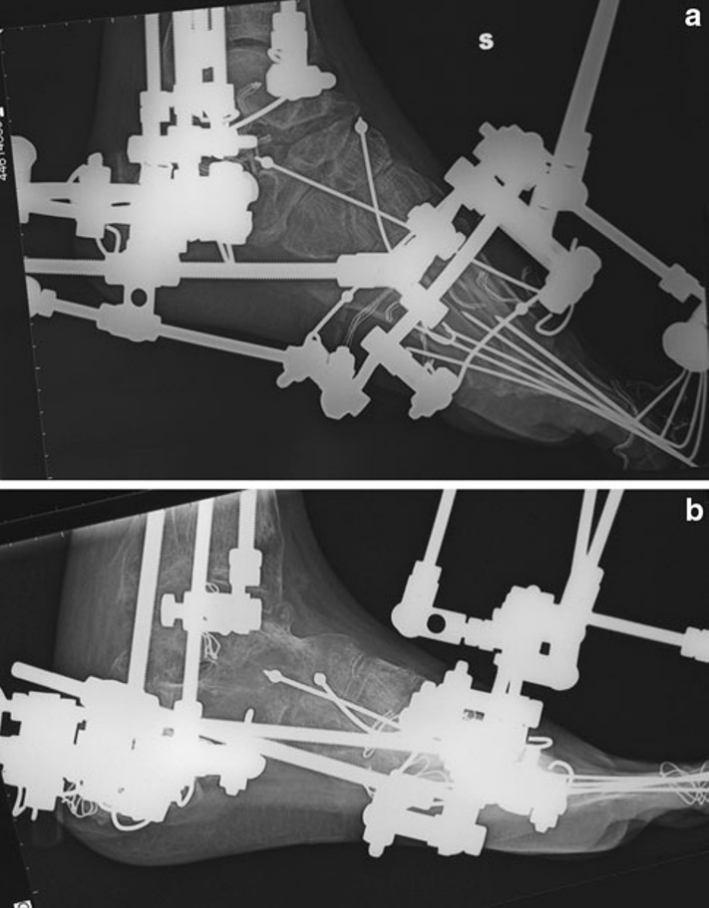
Plain X-ray (lateral view) of the foot and the ankle joint showing *V-*osteotomy and the use olives wires to prevent premature consolidation before distraction (a) and after correction (b)
